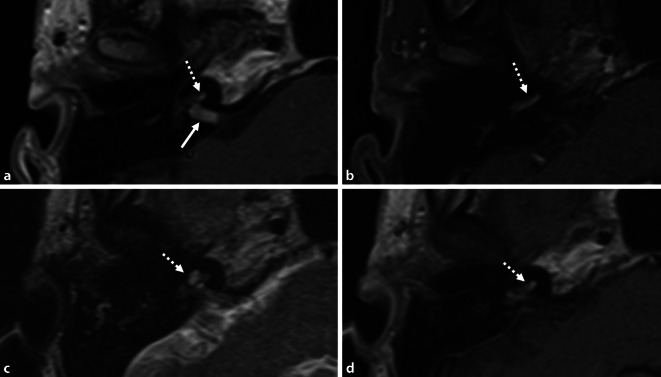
Patient 4 in Table 2. MRI (axial, T1‑w, + Gd). Transmodiolar ILS with resection of the intrameatal and, later, the intracochlear tumor parts. a,b Schwannoma in the IAC (→) and missed tumor in the cochlea (*dotted arrows*; 2013). c,d Situation 3 years after neurosurgical resection of the intrameatal and before resection of the intracochlear tumor parts
